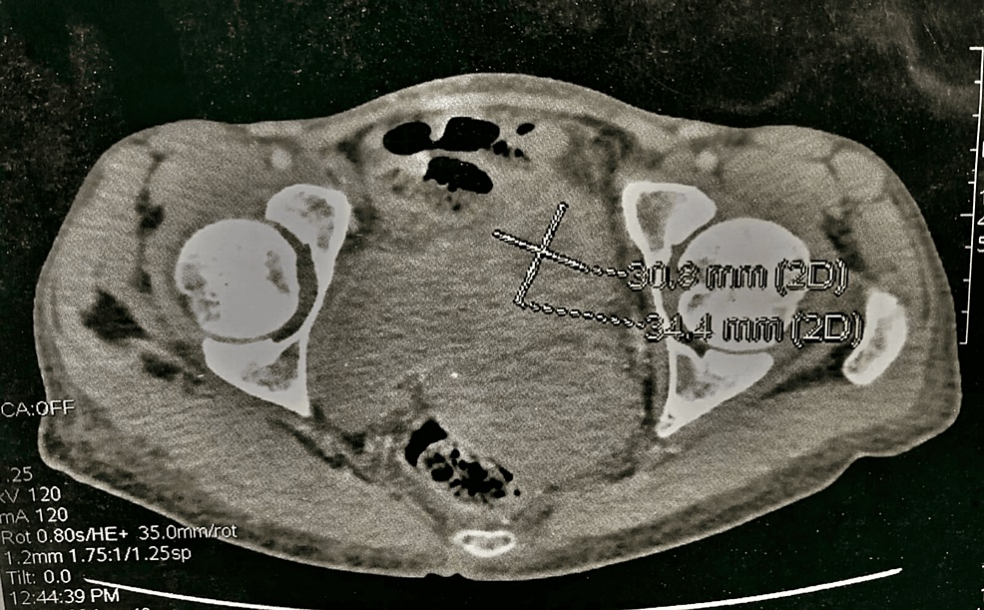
CT scan demonstrated a bladder mass situated at the dome
